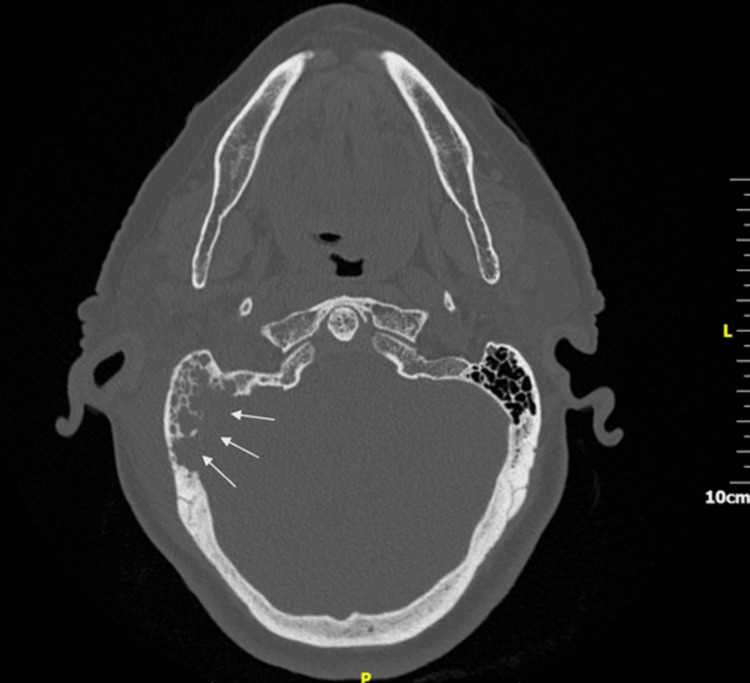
Axial plane of a CT scan temporal bones at the level of the mastoid showing complete opacification of the right middle ear and the mastoid air cells, along with extensive erosion of the mastoid cortical bone, posterior cranial fossa, and sigmoid sinus dural plate (arrows).
